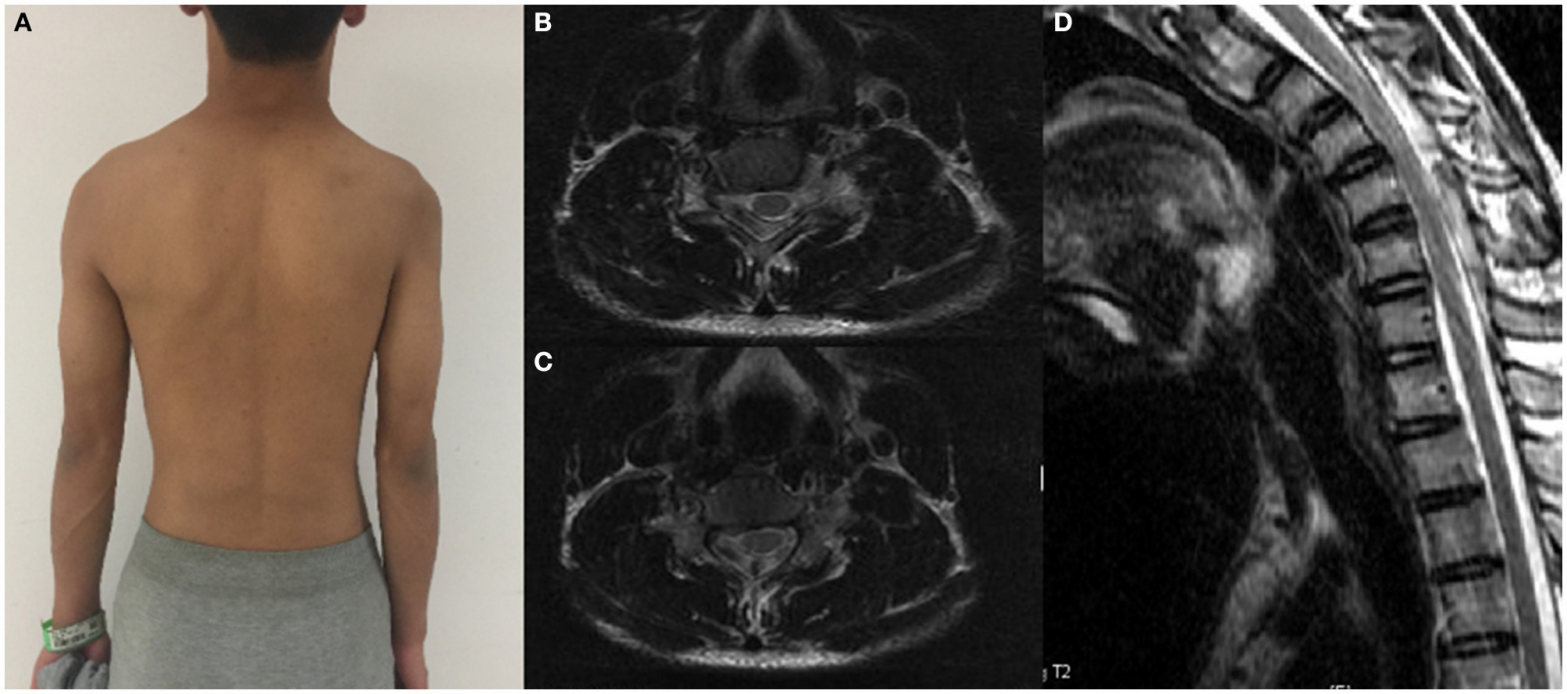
A patient with significant atrophy of the right proximal muscles of the upper extremity and his cervical flexion MRI. (A) Proximal muscle atrophy of right upper extremity. (B,C) Spinal cord flattening in horizontal view. (D) LOA in sagittal view.
